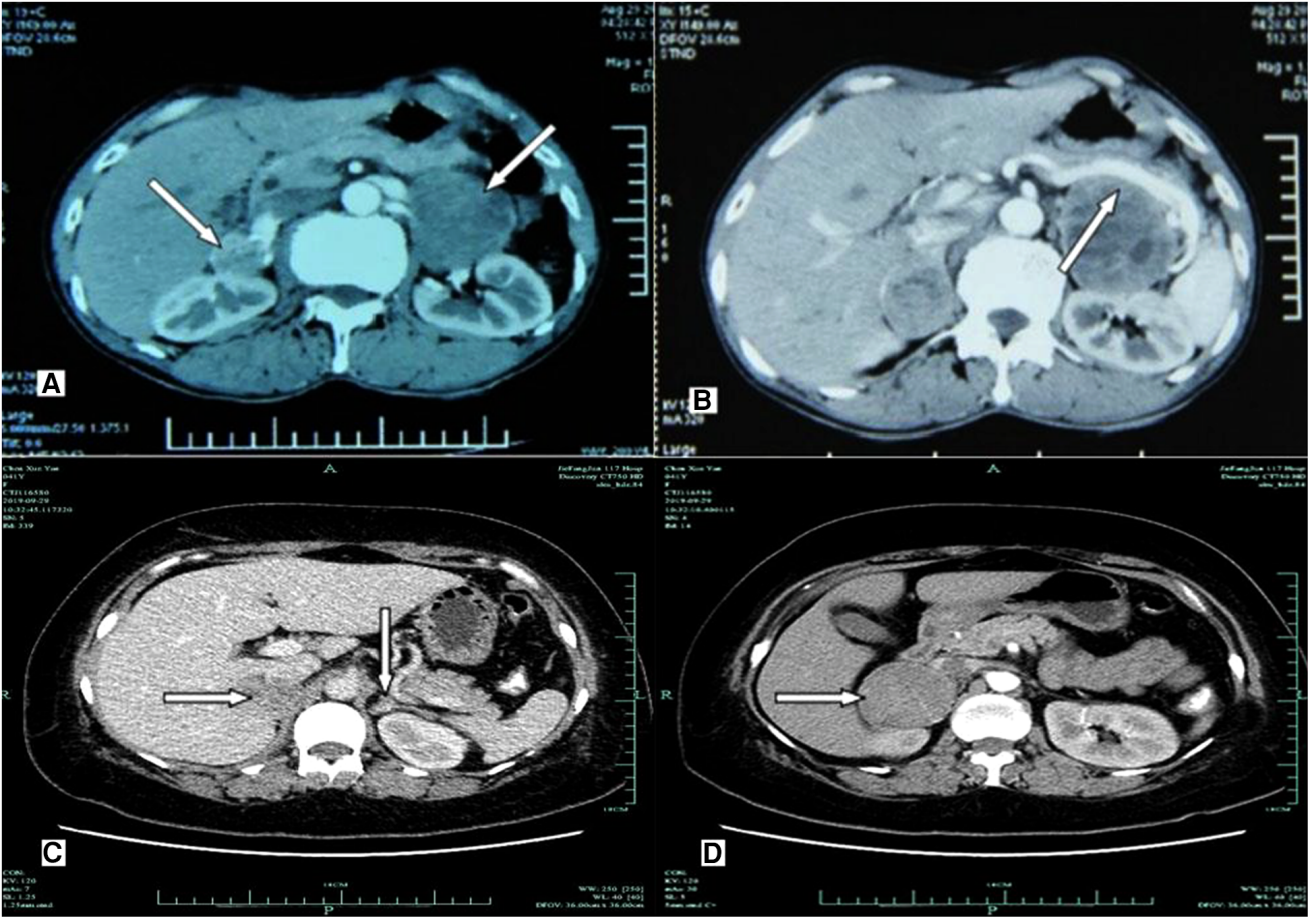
Imaging examination of computerized tomography scanning using ultravist 300 disclosed hypoechoic nodules in bilateral adrenal glands. (A,B) A 36-year-old male patient (P14; *RET*-C634Y) presenting with primary bilateral multifocal pheochromocytoma. (A) CT scan showing irregular enhancement of multiple nodules with intact capsule in both adrenal glands. (B) Contrast-enhanced CT imaging revealing inhomogeneous nodules with well-defined and heterogeneous enhancement in both adrenal glands (white arrows). The maximum diameter of tumors was 6.0 cm × 5.3 cm × 4.8 cm in the left, and the maximum diameter was 5.2 cm × 4.5 cm × 3.0 cm in the right, while having multiple low density lesions with no obvious enhancement, respectively. No areas of obvious necrosis or hemorrhage were observed. The splenic artery and vein showed compression and displacement (white arrow). (C,D) A 40-year-old female patient (P29; *RET*-C634Y) having bilateral pheochromocytoma. (C) Contrast-enhanced CT scanning showing that two primary inhomogeneous nodular and heterogeneous mild/moderate enhancement in the right adrenal (white arrows). A nodule (1.2 cm × 0.8 cm × 0.6 cm) with equal density in the left adrenal gland was enhanced to the same extent as the Normal adrenal tissue (white arrows), as possibility of recurrence of pheochromocytoma. (D) Larger one with clear boundary in the right adrenal was about 5.3 cm × 4.3 cm × 3.0 cm in diameter (white arrows).
